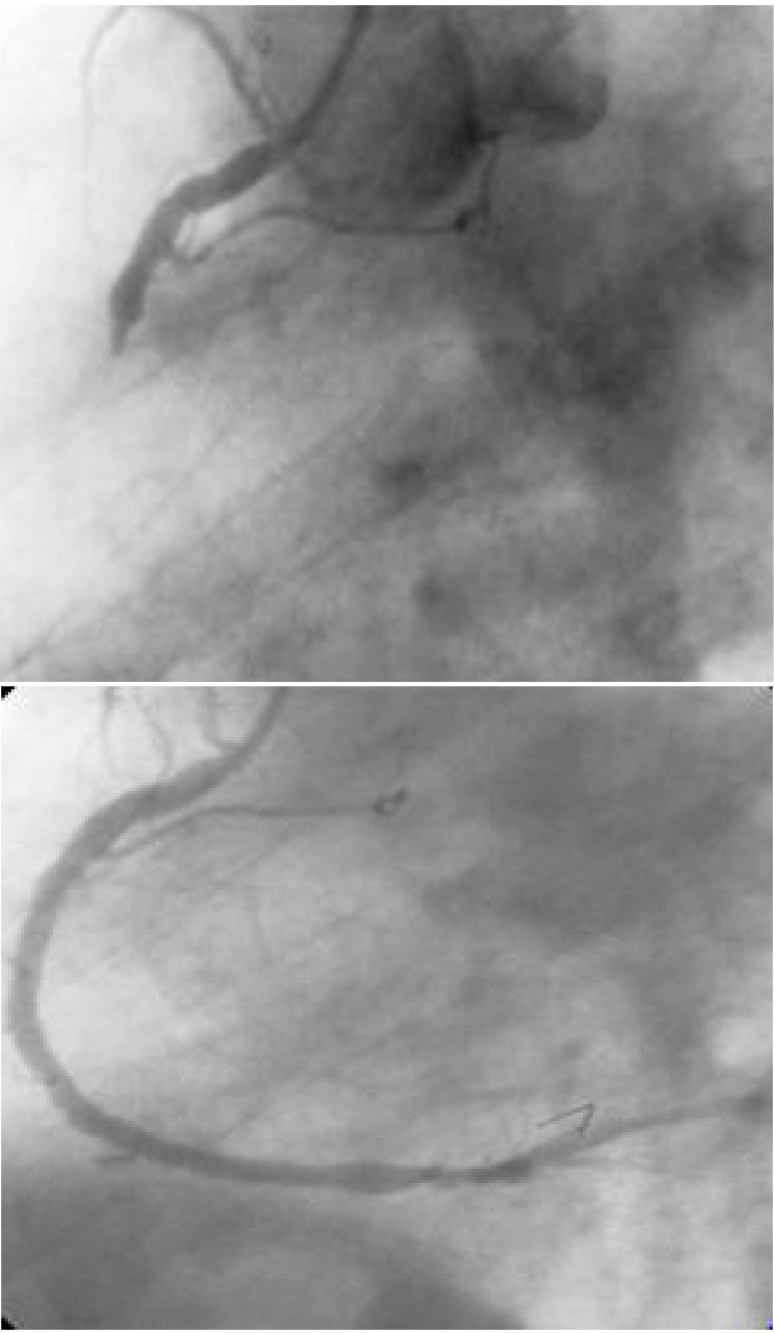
Myocardial blood flow quantification using contrast echocardiography; (ESC Guidelines 2009 on indications and usage of contrast echocardiography)
